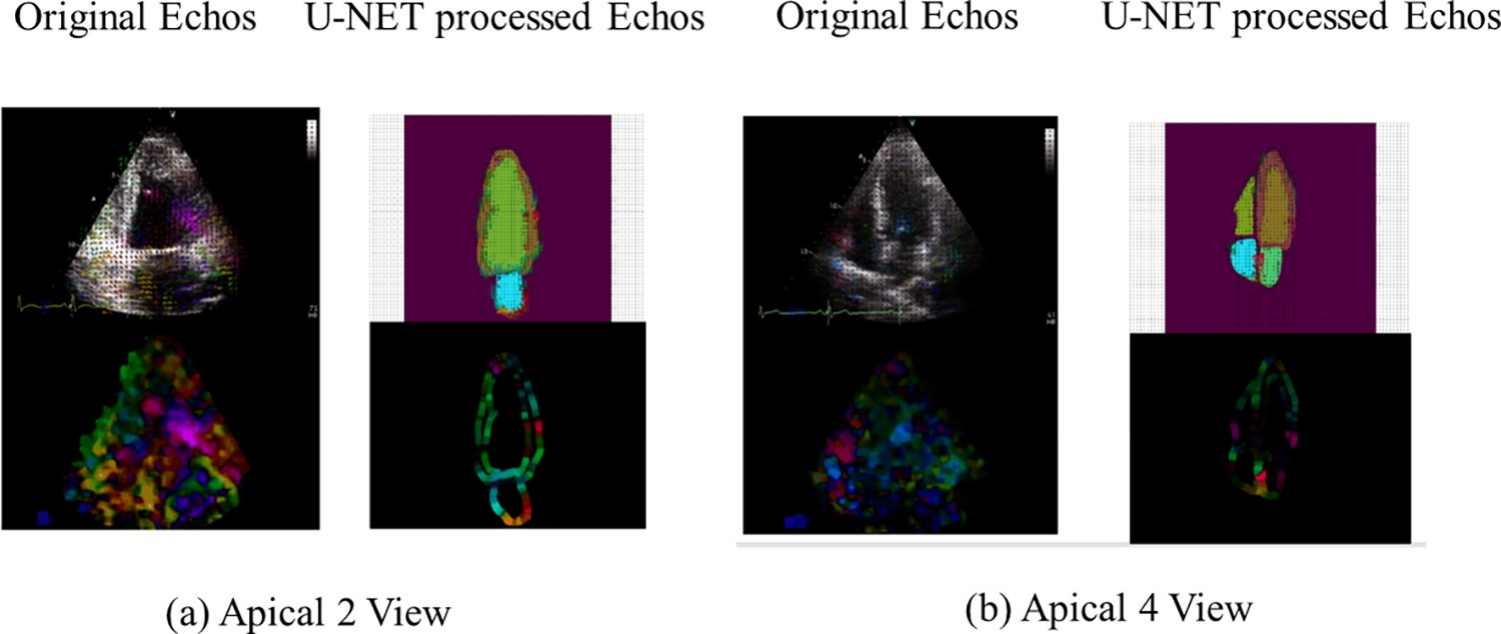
A comparative generated dense optical-flow frames of the original echocardiogram versus the U-Net processed echocardiogram. Representation includes the sample frame of input video, the generated optical flow frame, which are visually enhanced with colour arrows to indicate the direction and magnitude of motion, for both (a) Apical 2 View and (b) Apical 4 View.
